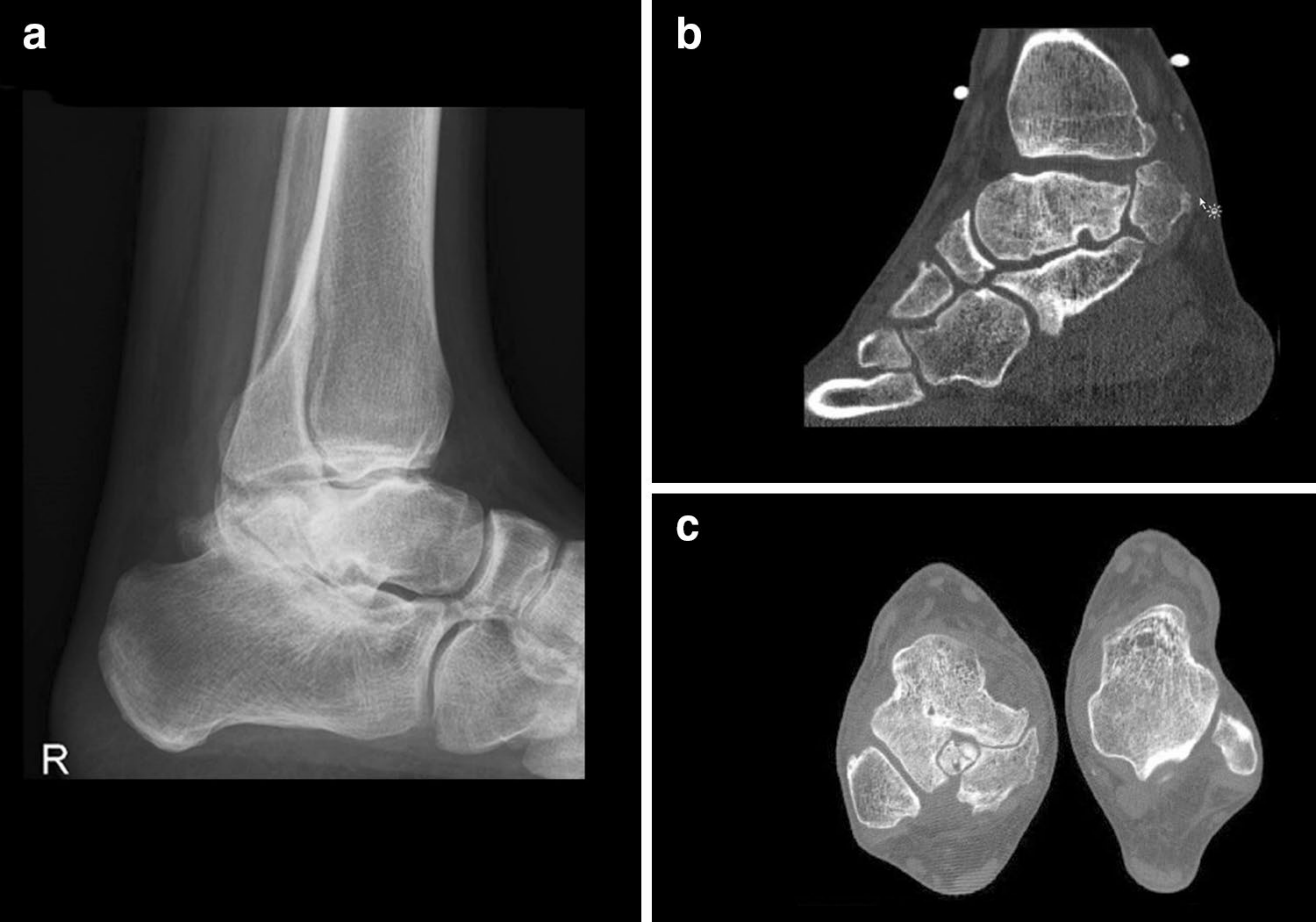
Radiographic images case 3. a Weight-bearing lateral radiograph showing a deformed talus and osteoarthritic changes in the subtalar joint. b Sagittal CT image showing a flattened talar dome and talus bipartitus. c Axial CT image showing a talus bipartitus at the right side. The left talus reveals a normal morphology
